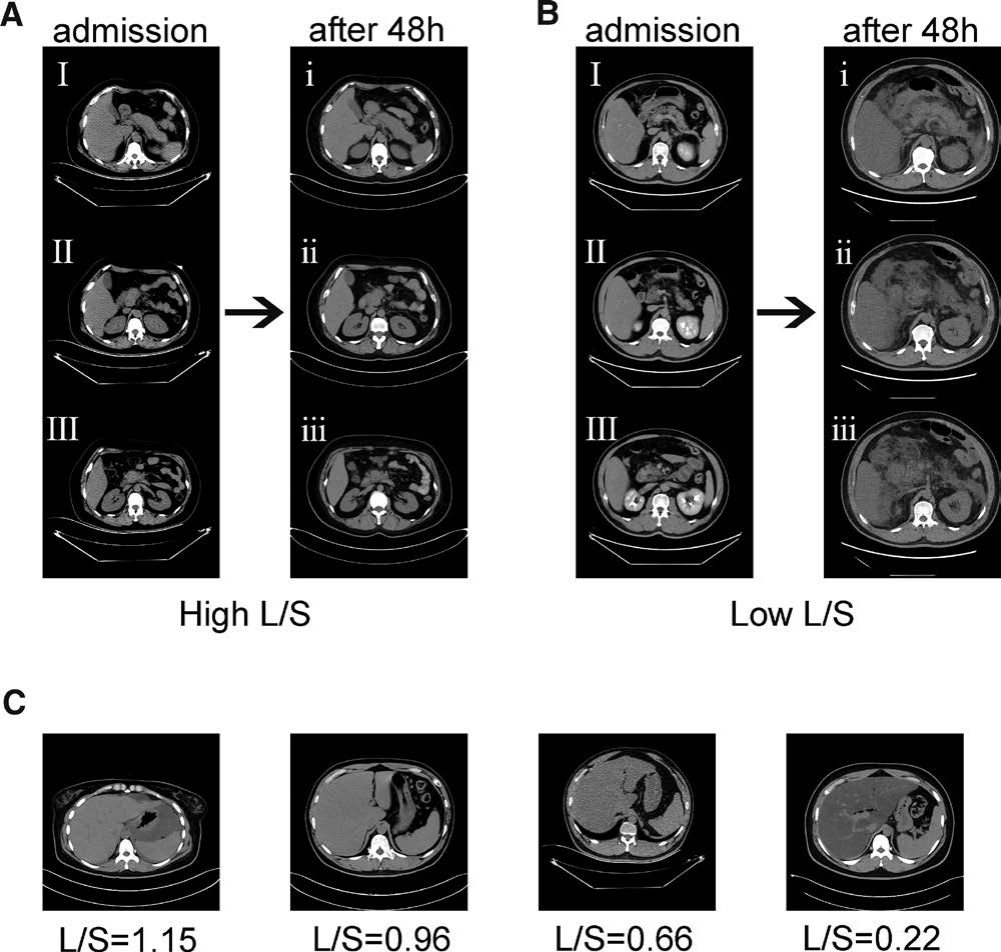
Representative cases of HTG-AP on CT scans. (A) Changes in pancreatic CT findings in patients with a high L/S ratio. (B) Changes in pancreatic CT findings in patients with a low L/S ratio. (C) CT scans of varying degrees of fatty liver in patients with HTG-AP. I and i, body and tail of pancreas; II and ii, head of pancreas; III and iii, uncinate process of pancreas.
